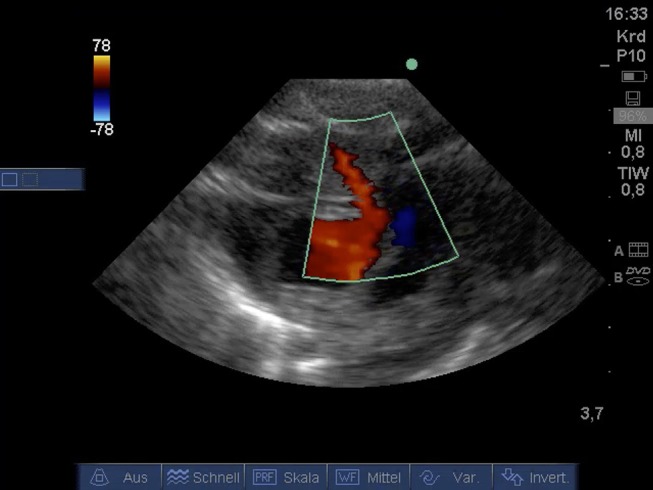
Color Doppler image of coronary artery from PSAX (age: 32 h, weight: 1.36 kg). CA, coronary artery; PSAX, parasternal short axis.
